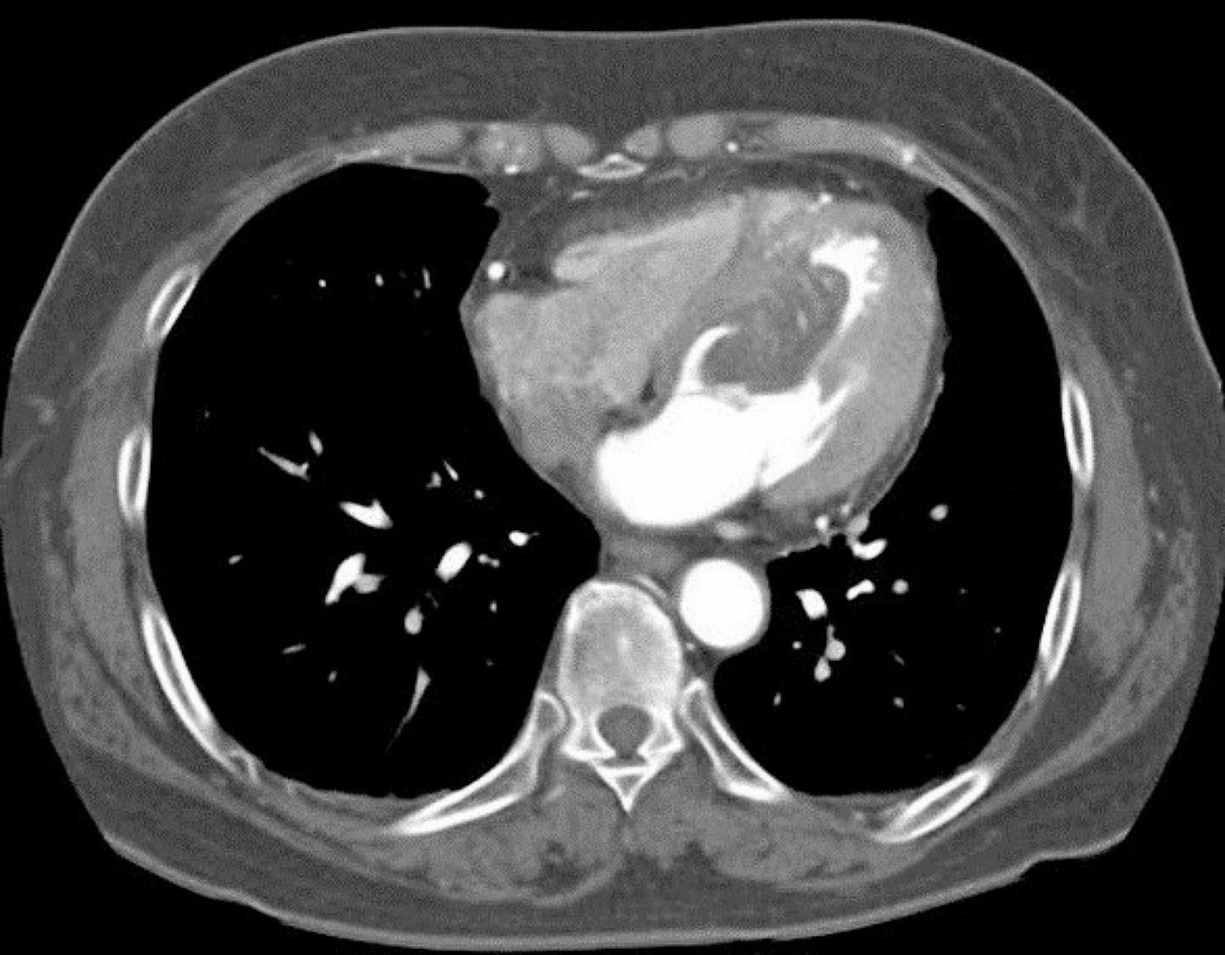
Initial coronary computed tomography angiography showing a mass adherent to the septum in the left ventricular cavity
